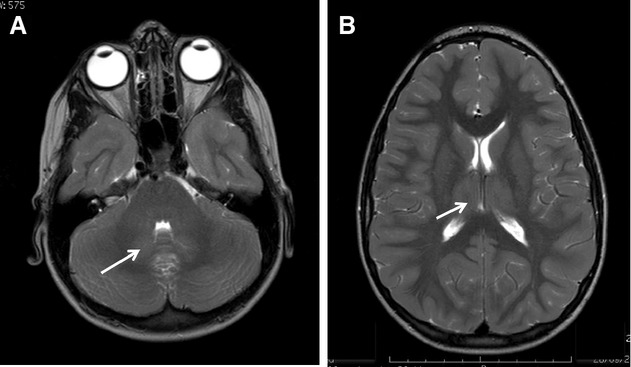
Increased T_2_ signal intensities in dentate nuclei and thalamic regions in brain magnetic resonance imaging (MRI) associated with *POLG* mutations. Axial T_2_-weighted MR images from patient 4, who had p.W748S;E1143G + G1205E mutations. There is mildly increased signal intensity (SI) in the region of the dentate nuclei (A, arrow) and diffuse high SI in both thalami (B, arrow). Increased SI in the same areas was also seen on the fluid-attenuated inversion recovery (FLAIR) images but no abnormal findings were detected on the T_1_-weighted images. No other lesions were seen in this patient and the findings were very similar between the scan at presentation (shown) and a scan done 3 months later.
